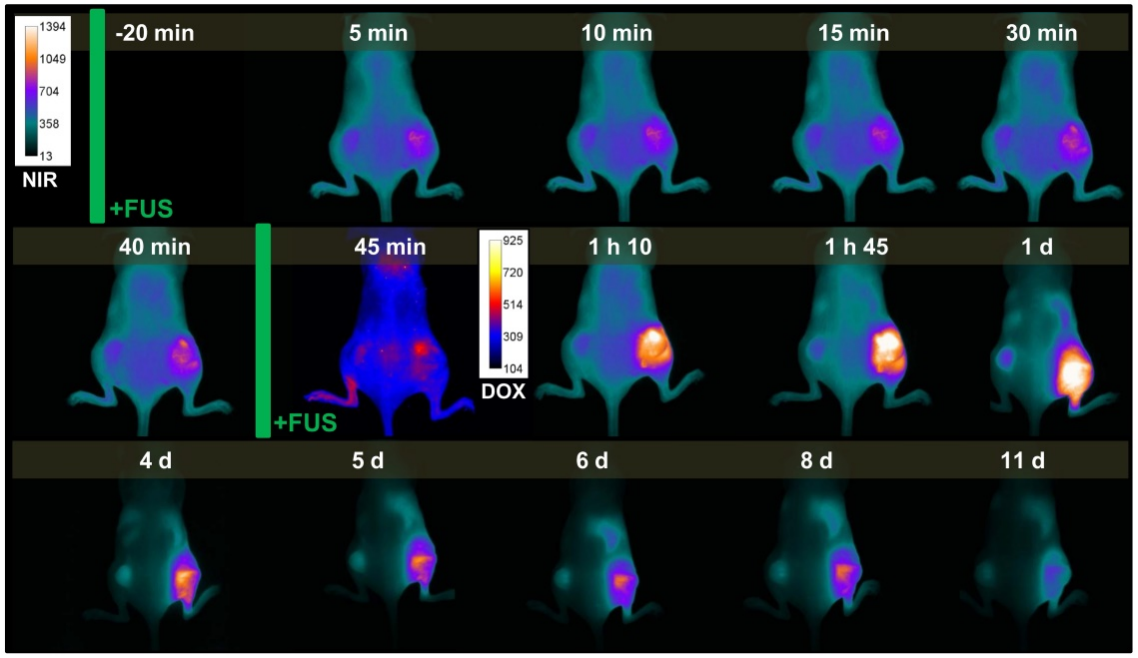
Representative example of near-infrared (NIRF) and doxorubicin fluorescence imaging during the dual-tumour study. Time points are pre/post-injection and focused ultrasound (FUS) treatments are indicated by green bars. Right tumour received FUS, while left tumour did not receive FUS. NIRF images are coloured cyan-yellow, doxorubicin are indigo-red.
